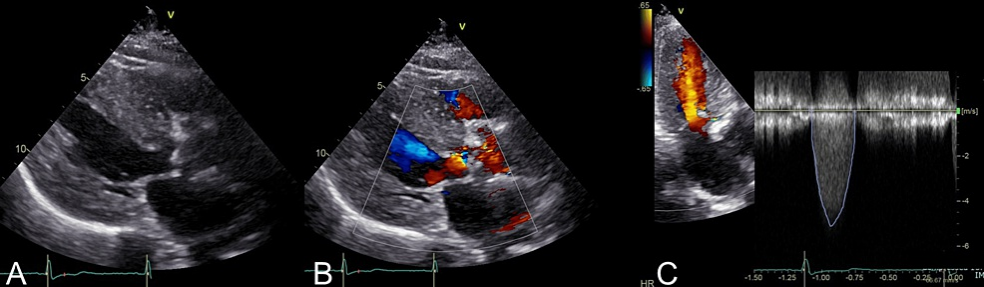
Preoperative transthoracic echocardiography of the present case A) Left ventricular long-axis view (B mode); B) left ventricular long-axis view (Color Doppler mode); C) continuous wave Doppler of aortic valve blood flow (apical 3-chamber view) B-mode showed an aortic valve with calcification and restricted opening. Color Doppler mode showed accelerated blood flow due to aortic valve stenosis. Continuous wave Doppler of aortic valve blood flow revealed the maximum velocity was 5.1 m/sec.
